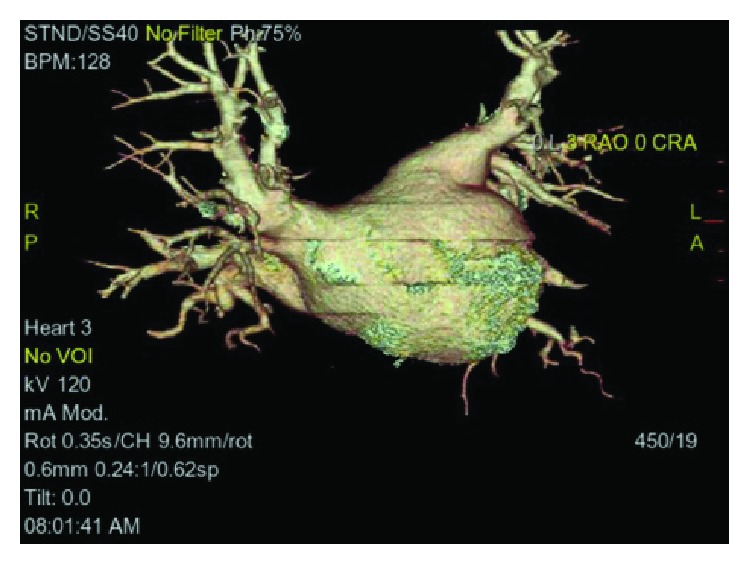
CTA of the pulmonary veins at anteroposterior (AP) view showing there is no left atrial appendage.
